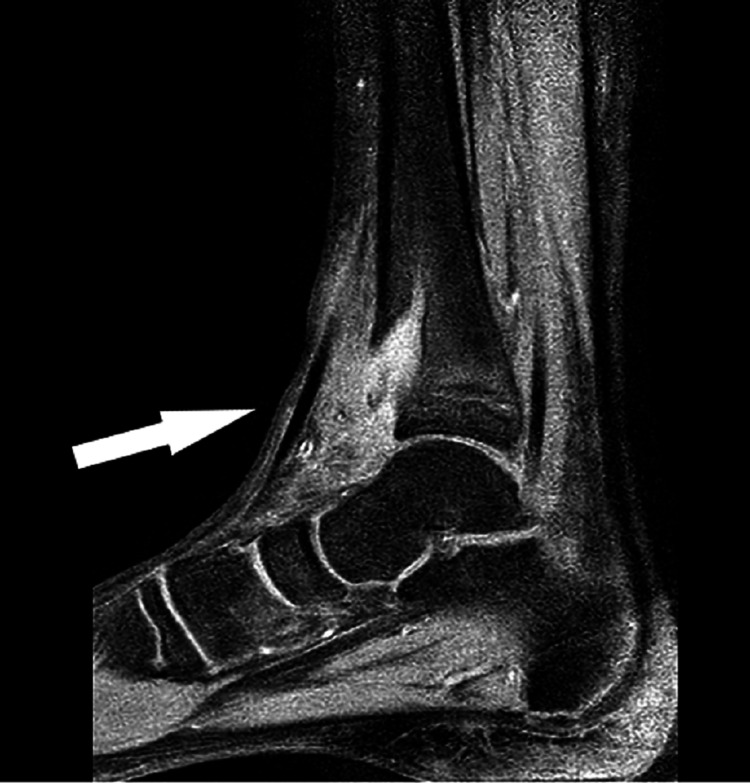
Another MRI image of the same abscess showing involvement of physis and extension into the metaphysis
